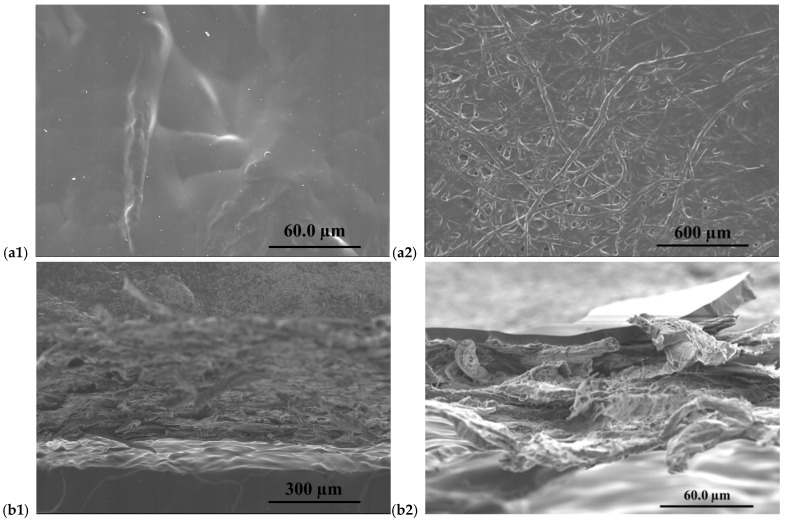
SEM images of surface and cross-section of CTA-based filter paper–PIM (a1,b1), and PVC-based filter paper–PIM (a2,b2).
